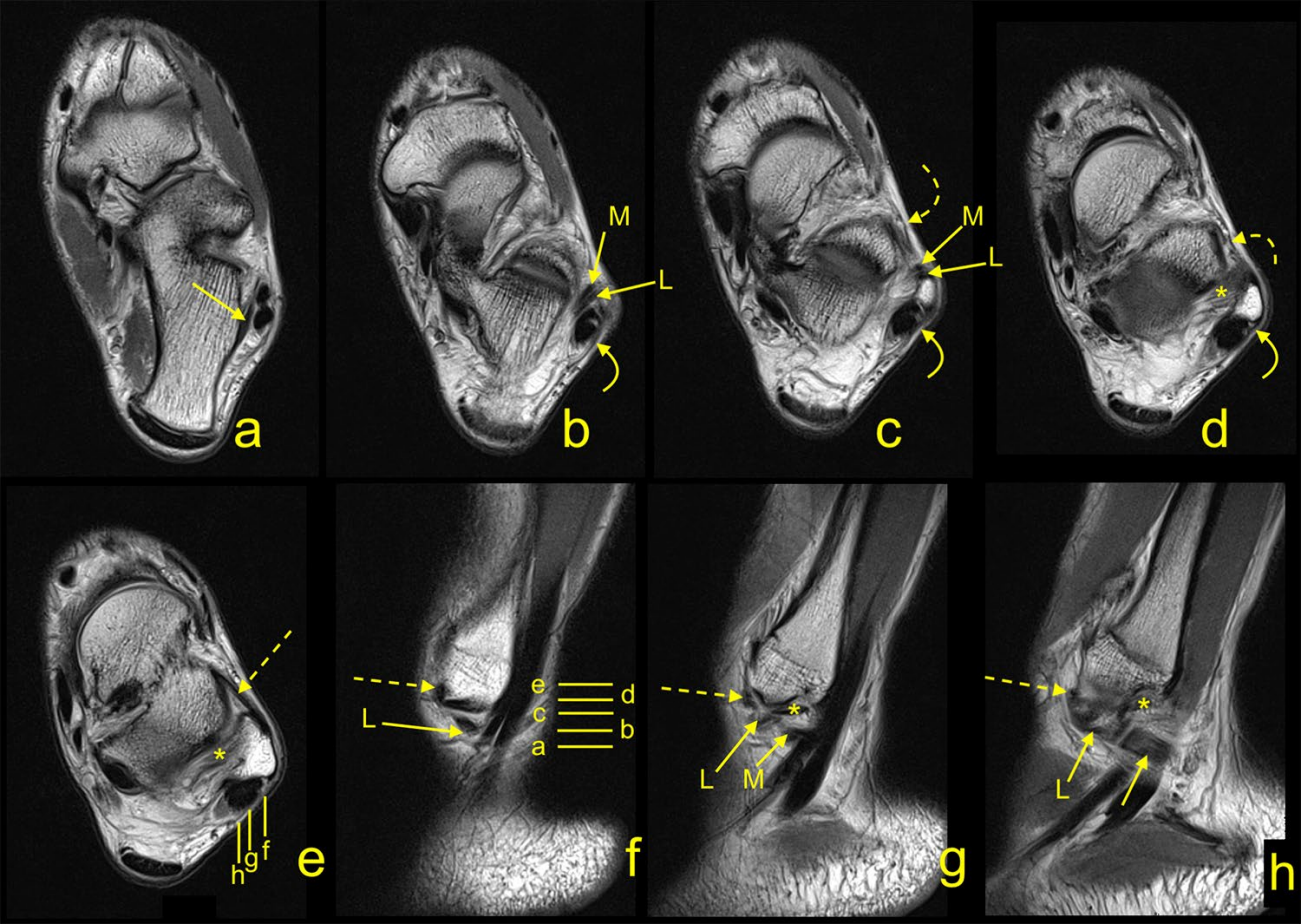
A 33-yeard-old patient with suspicion of an osteochondral lesion of the talus, which was not confirmed on the MRI. The communications present in relation to the lateral malleolus. The CFL (straight arrow), the ATFL (dashed arrow), the SPR (curved arrow), the IER (curved dashed arrow), and the PTFL (*). On figure (b, c, f, g and h) two fascicles of the CFL are visible: L- the L-CFL and M- the M-CFL. Levels of cross-sections are labeled on figures (e) and (f).
